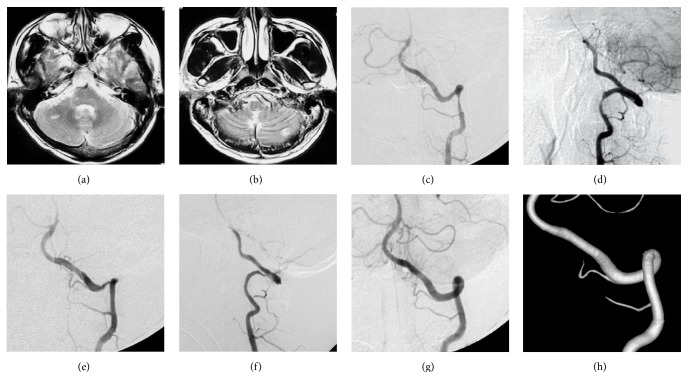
MRI ((a) and (b)) showed multiple infarcts in the bilateral cerebellum especially in the right cerebellar hemisphere. Frontal and lateral DSA ((c) and (d)) of left VA angiogram showed the dissection in the segment of V3. Stenosis was the major appearance. Although the left VA was the nondominant one, the embolus is easy to escape and enter into both the right and left AICA (more into the right one) due to neck motion and the characteristics of anatomy of right AICA (the VA distal to the dissection, inferior segment of BA, and the initial segment of right AICA are almost in a line). Frontal and lateral DSA showed the stenosis disappeared after stenting ((e) and (f)). 2-dimensional and 3-dimensional DSA ((g) and (h)) showed the anatomical healing of the involved VA when following up. MRI: magnetic resonance image; DSA: digital subtraction angiography; VA: vertebral artery; AICA: anterior inferior cerebellar artery; BA: basilar artery.
